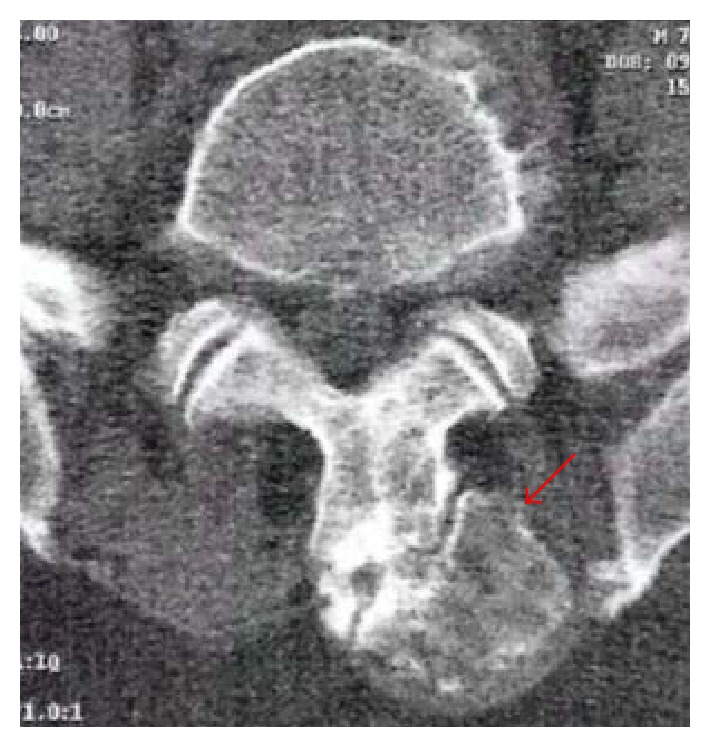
CT scan. Axial view showing a well-limited mass with a cartilage cap arising from the spinous process of L5 lateralized to the left (arrow).
